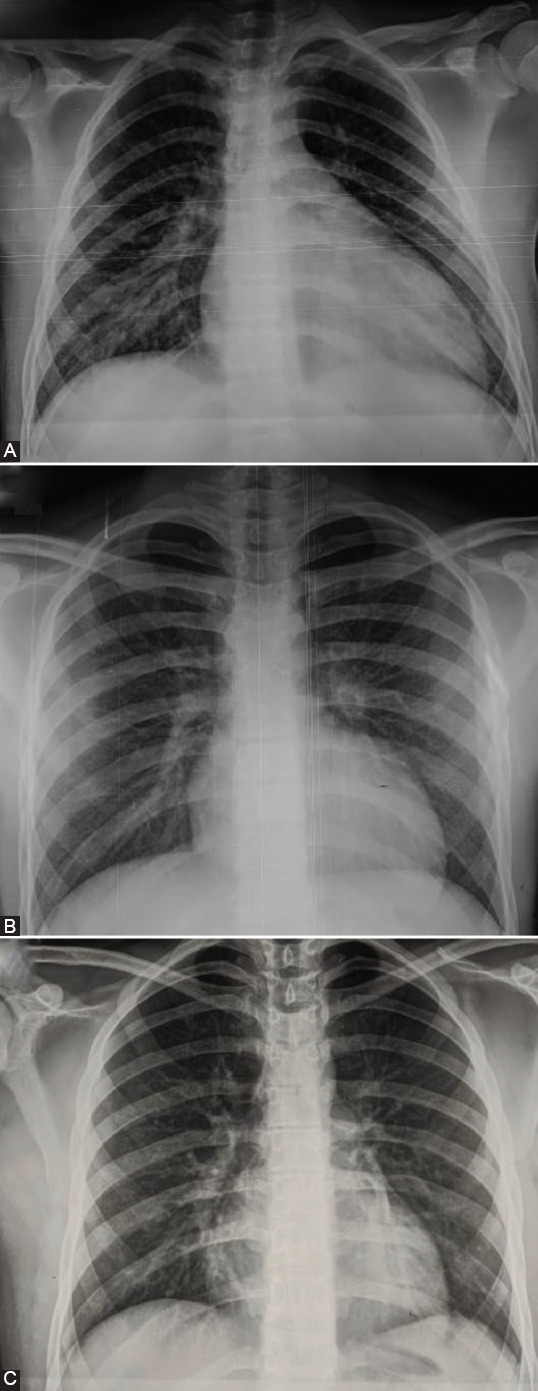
Chest radiography at the admission. (A) 1° patient: Enlarged cardiac shadow, diffusely accentuated pulmonary interstitium, bilateral pleural effusion; (B) 2° patient: Normal finding; (C) 3° patient: Diffusely accentuated pulmonary interstitium.
